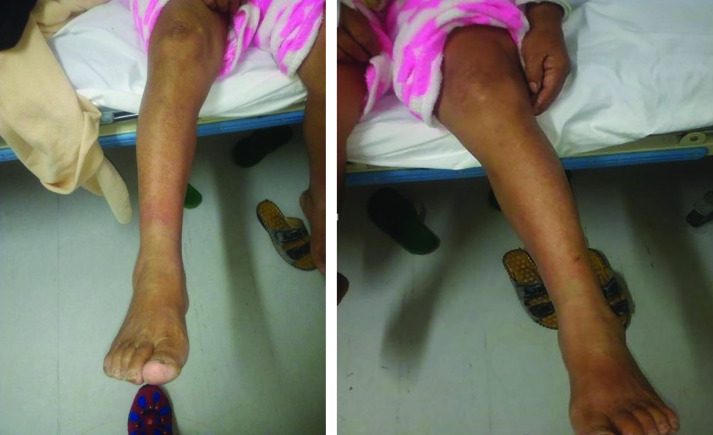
Chest radiographic image
